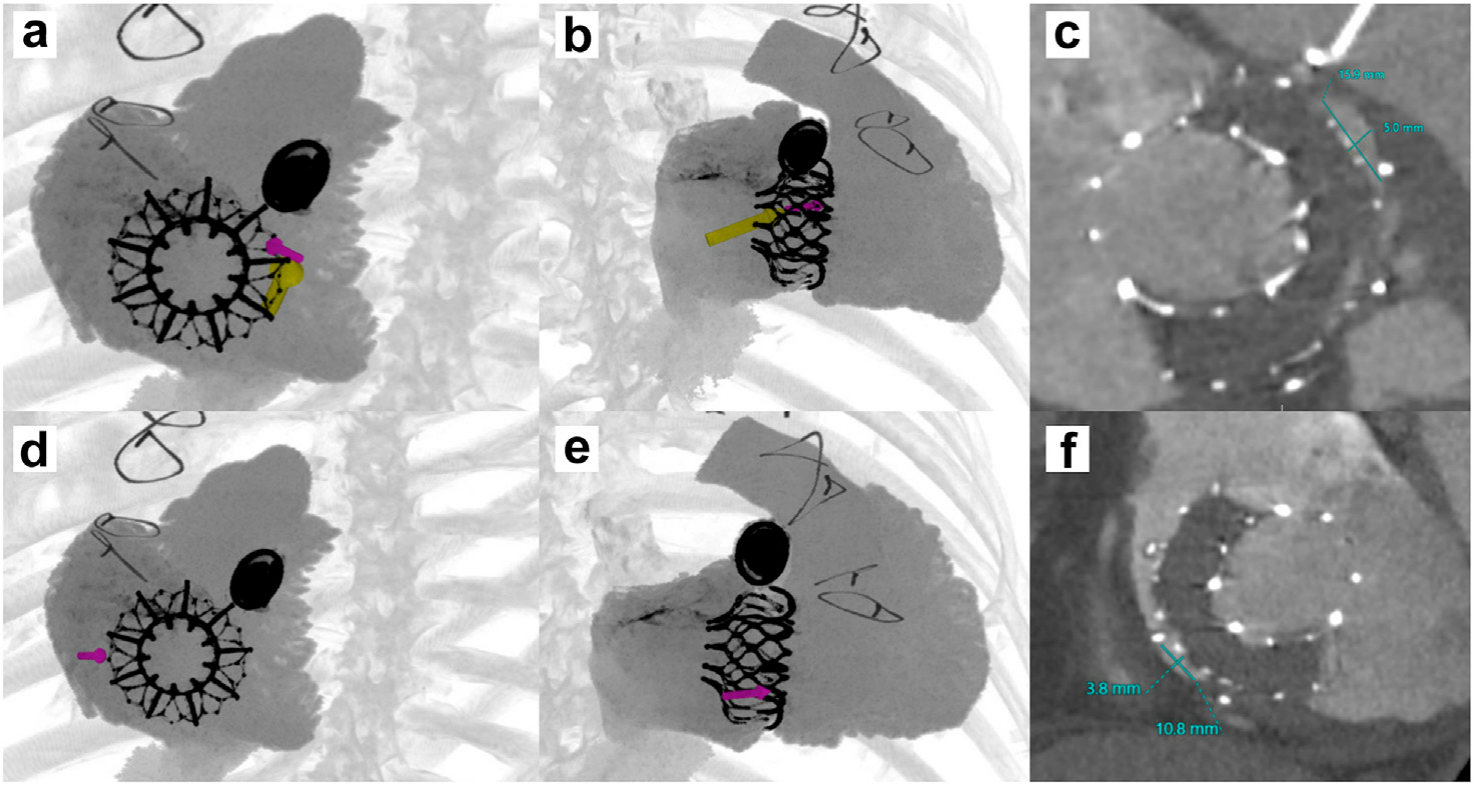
Preoperative cardiac computed tomography. (a-c) Antero-septal PVL at 2 o’clock and (d-f) postero-lateral PVL at 8 o’clock. Abbreviation: PVL, paravalvular leak.
